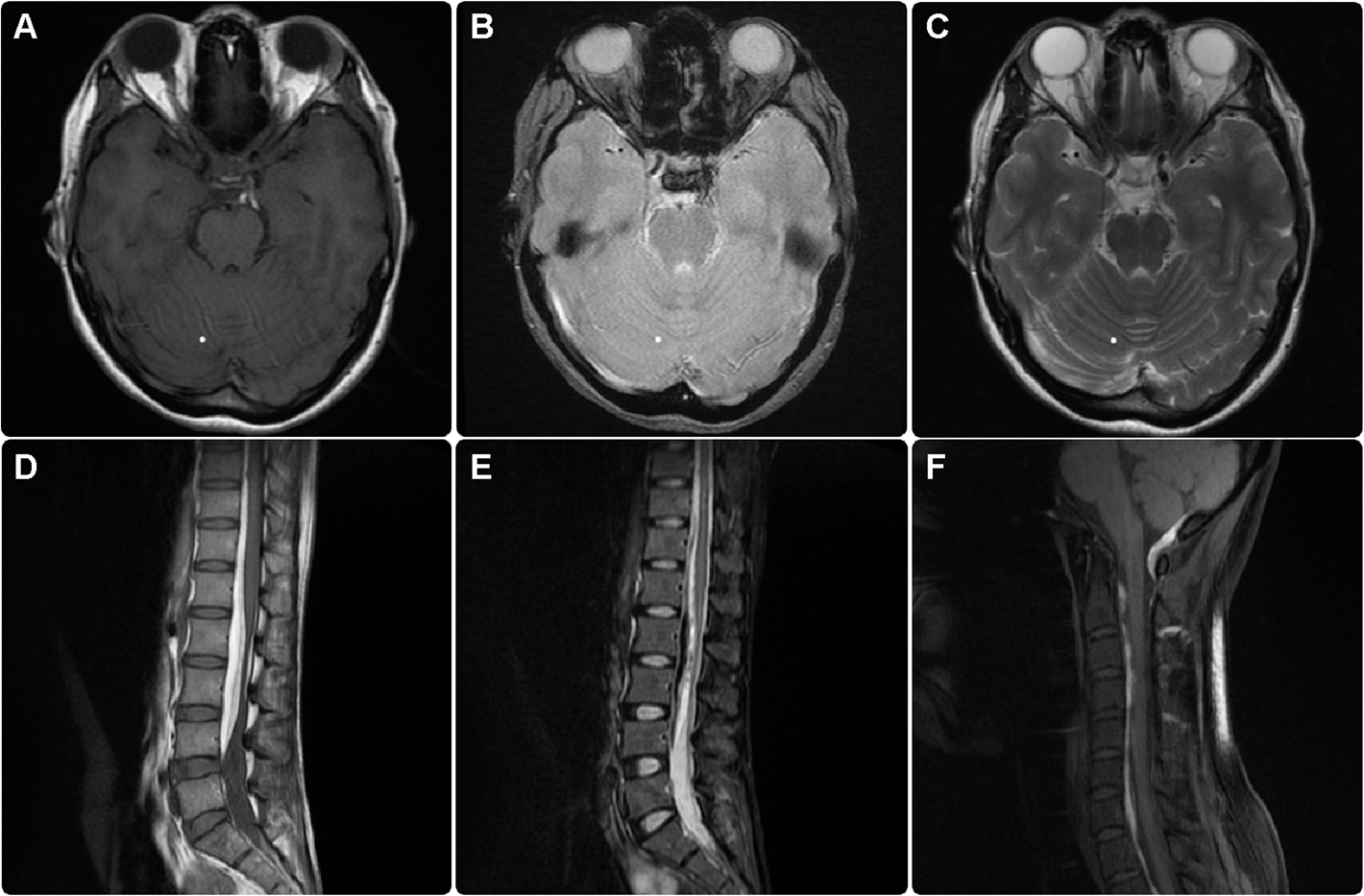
Multi-sequential magnetic resonance imaging (MRI) of the brain: axial T1-weighted (a), gradient (b), and T2-weighted (c) images showing a small area of extra-axial hyperintensity in the T1 image, and iso- to low-signal intensity in the T2 image, and a susceptibility artifact located at the anterior left tentorial edge, medial to the uncus, representing a subacute to chronic subdural hemorrhage in the gradient image. Multi-sequential MRI of the spine: sagittal T1-weighted (d), short tau inversion recovery (STAIR) of the lumbar spine (e), and T1-weighted fat saturation (f) images of the cervicothoracic spine showing massive extradural (subdural) bleeding presenting as predominantly high signal intensity on T1 (d) and predominantly low signal intensity on T2 STAIR (e) images, which show blood collection along the spinal canal extending from the posterior foramen magnum down to the lumbar spine, thus representing subdural hemorrhage
